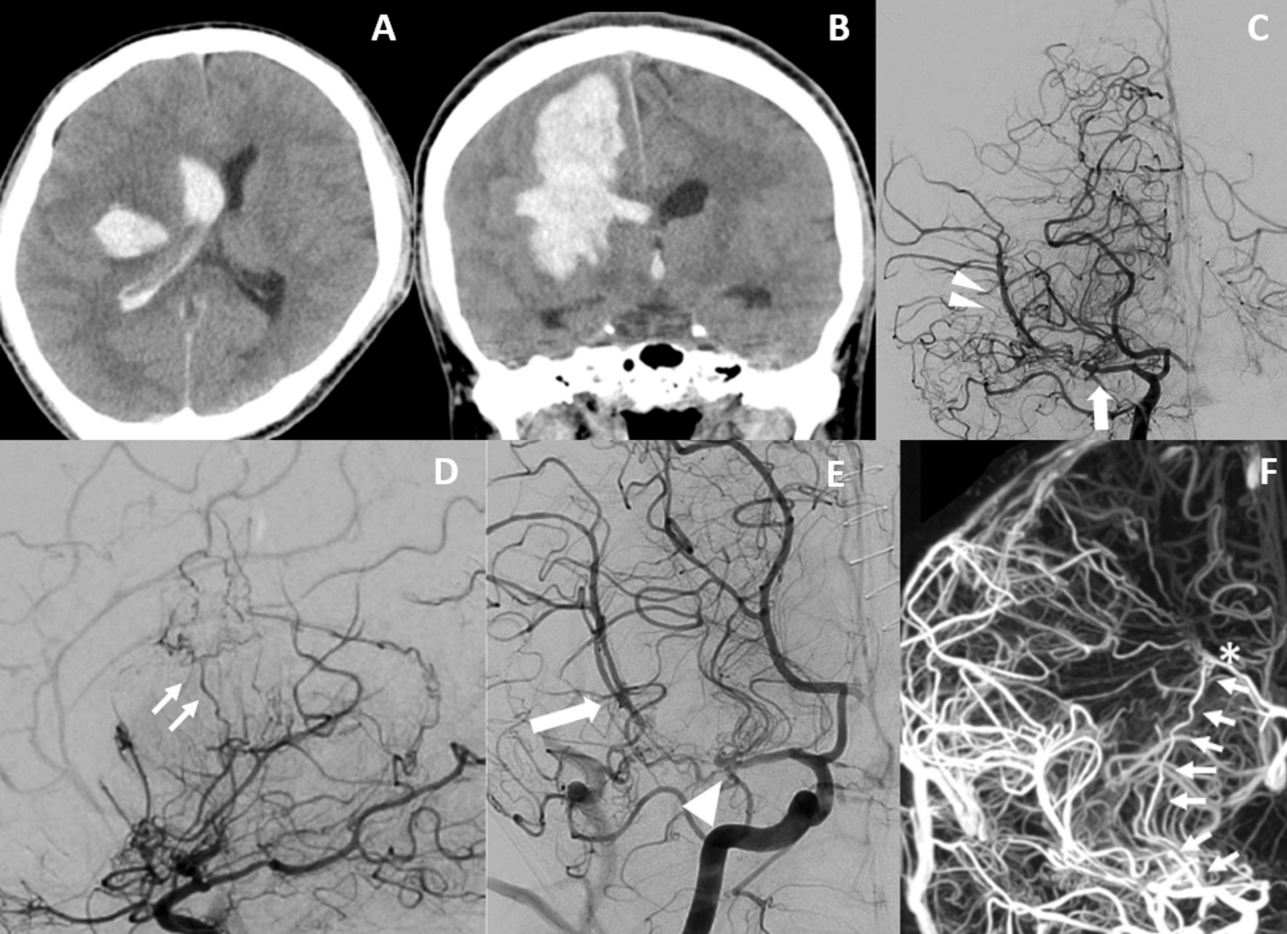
CT and artery angiography on admission A, B: Head CT on admission (A: axial B: coronal). About 66 ml of cerebral hemorrhage from the right putamen to the frontoparietal cortex and　hydrocephalus due to perforation into the lateral cerebral ventricle. C-F: Right internal carotid artery angiography (C: anterior-posterior view D: lateral view E: anterior-posterior view capturing the characteristics of T-MCA F: Vaso CT). Vascular occlusion in the right MCA M1 portion and a vascular network in the surrounding area (C: arrow). There was no narrowing of the terminal segment of the internal carotid artery and distal MCA to the steno-occlusive lesion (C: double arrowhead, E: arrowhead). Periventricular anastomosis was observed between the dilated LSA (F: arrow) and the medullary artery (E: double arrow F: arrow, asterisk).
